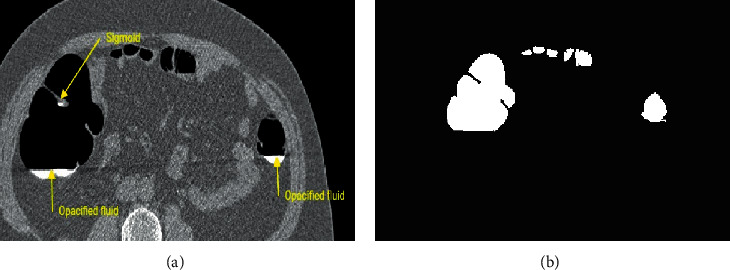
(a) Original CT image (axial slice). (b) Segmentation of colon air pockets.
